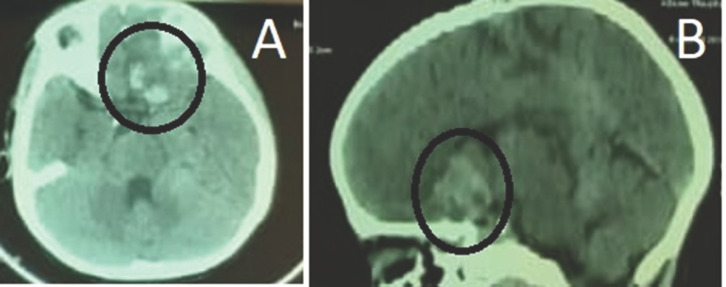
Scanner cérébral en coupe axiale (A) et sagittale (B) sans injection du produit de contraste mettant en évidence une hyperdensité spontanée frontale gauche entourée d'un oedème péri-lésionnel en faveur d'une hémorragie cérébrale intraparenchymateuse (cercle noir) Non contrast brain Computed Tomography scan in axial (A) and sagittal (B) cut, showing left frontal spontaneous hyperdensity with perilesional edema suggesting brain intraparenchymal haemorrhage (black circle)
